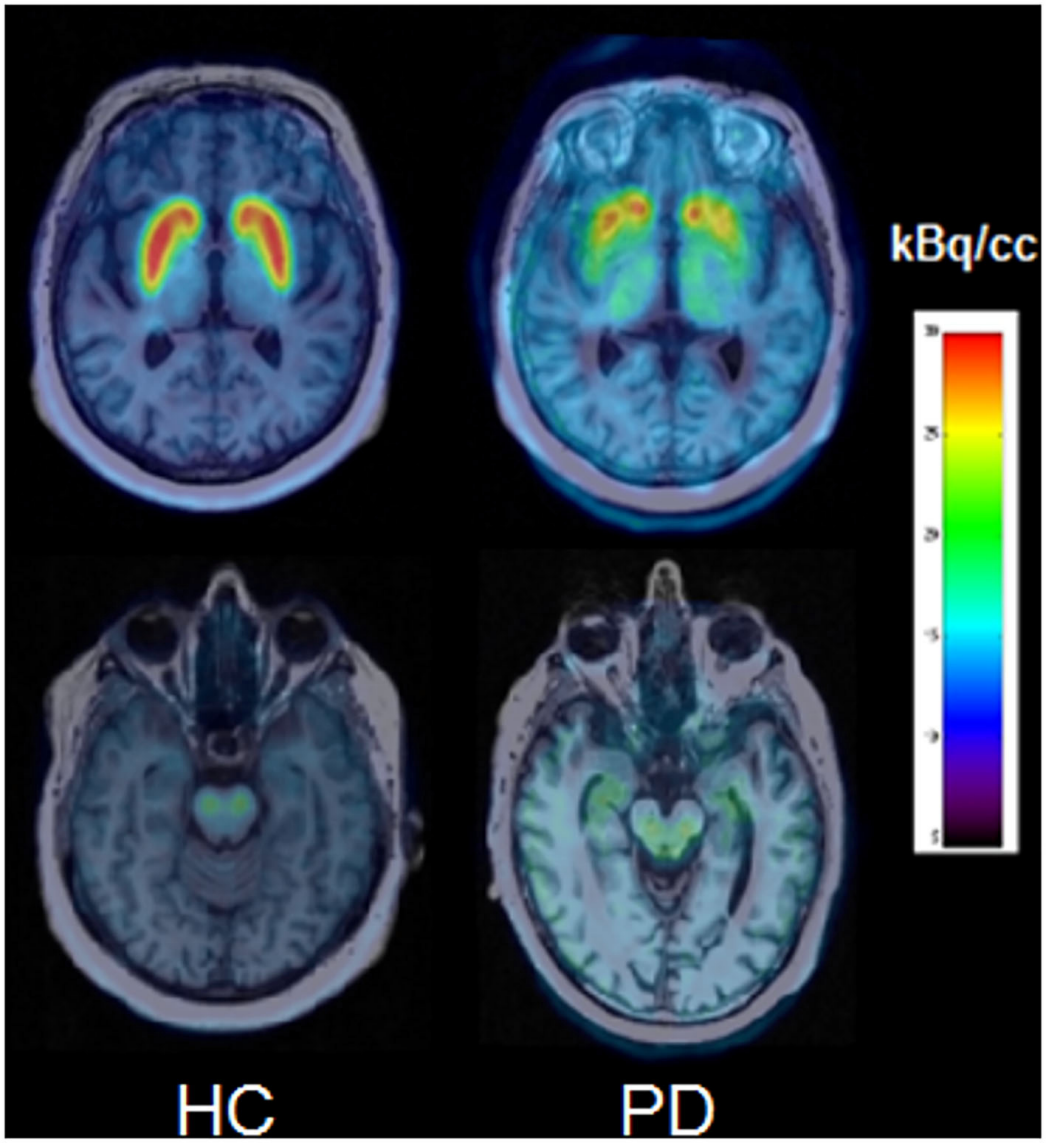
PET/MRI axial slices obtained between 30 and 40 min post-injection for a healthy control (HC) and a PD patient at the level of striatal nuclei (top) and midbrain (bottom).
